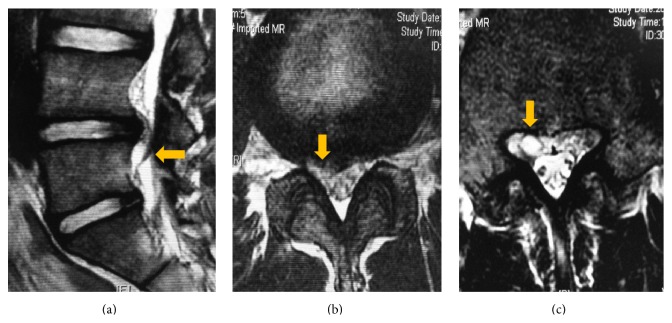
Preoperative magnetic resonance imaging: (a) sagittal T2-weighted image, (b) axial T2-weighted image through the L4-L5 disc, and (c) axial T2-weighted image through L5 pedicle demonstrate the extradural cystic lesion with high signal intensity, communicating with the corresponding intervertebral disc (arrows).
